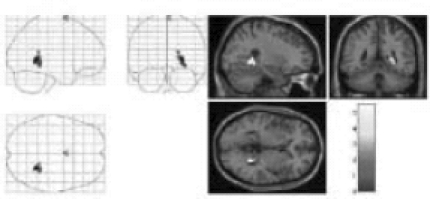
Right Parahippocampal Gyrus Activation in the subject with telepathic ability [Mr. GS], while performing a successful telepathic task. On the left hand side, the activation is superimposed on a glass brain and on the right hand side, the activation [yellow] is superimposed on a structural MR image
